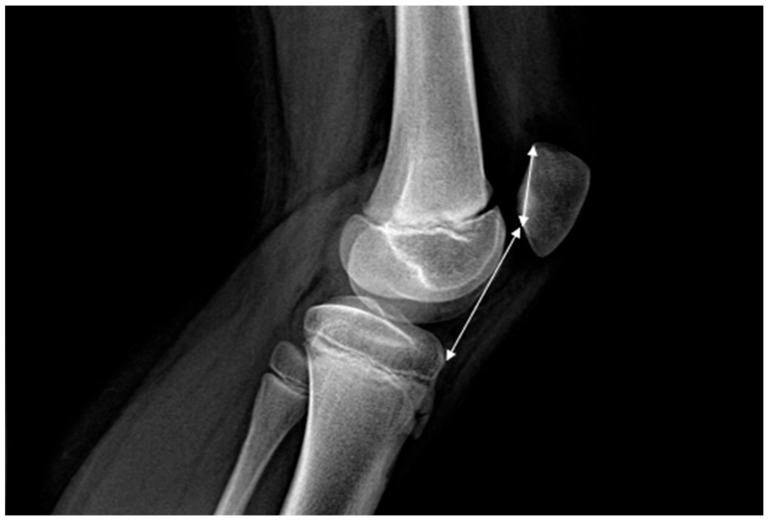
CDI represents the ratio between the length of the patellar articular surface and the distance from the anterior angle of the tibial plateau to the most inferior point of the patellar articular surface, measured on a lateral radiograph with the knee in 30 degrees of flexion.
